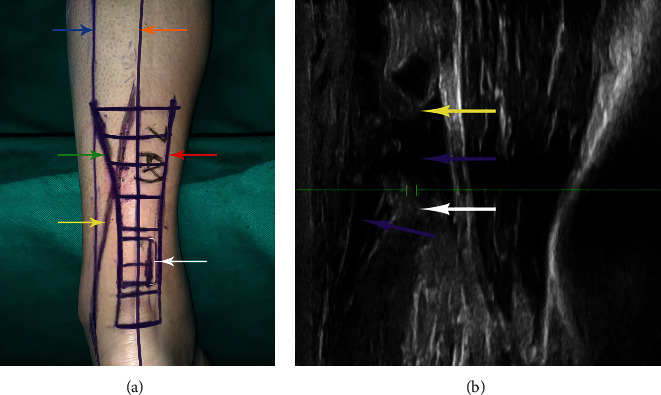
(a) The anatomical structure was located preoperatively by body surface localization and ultrasonography. The blue arrow shows the course of the small saphenous vein and sural nerve drawn through the body surface markers. The yellow arrow shows the exact course of small saphenous vein and sural nerve located by ultrasonography. The orange arrow represents the midline of the Achilles tendon measured by ultrasonography. The red arrow shows the medial edge of the Achilles tendon on ultrasonography. The green arrow shows the lateral edge of the Achilles tendon on ultrasonography. The white arrow shows the broken end of the Achilles tendon on ultrasonography. (b) Ultrasonography image of ruptured Achilles tendon in sagittal view. The yellow arrow shows proximal Achilles tendon stump. The white arrow shows distal Achilles tendon stump. The purple arrow shows hematoma.
